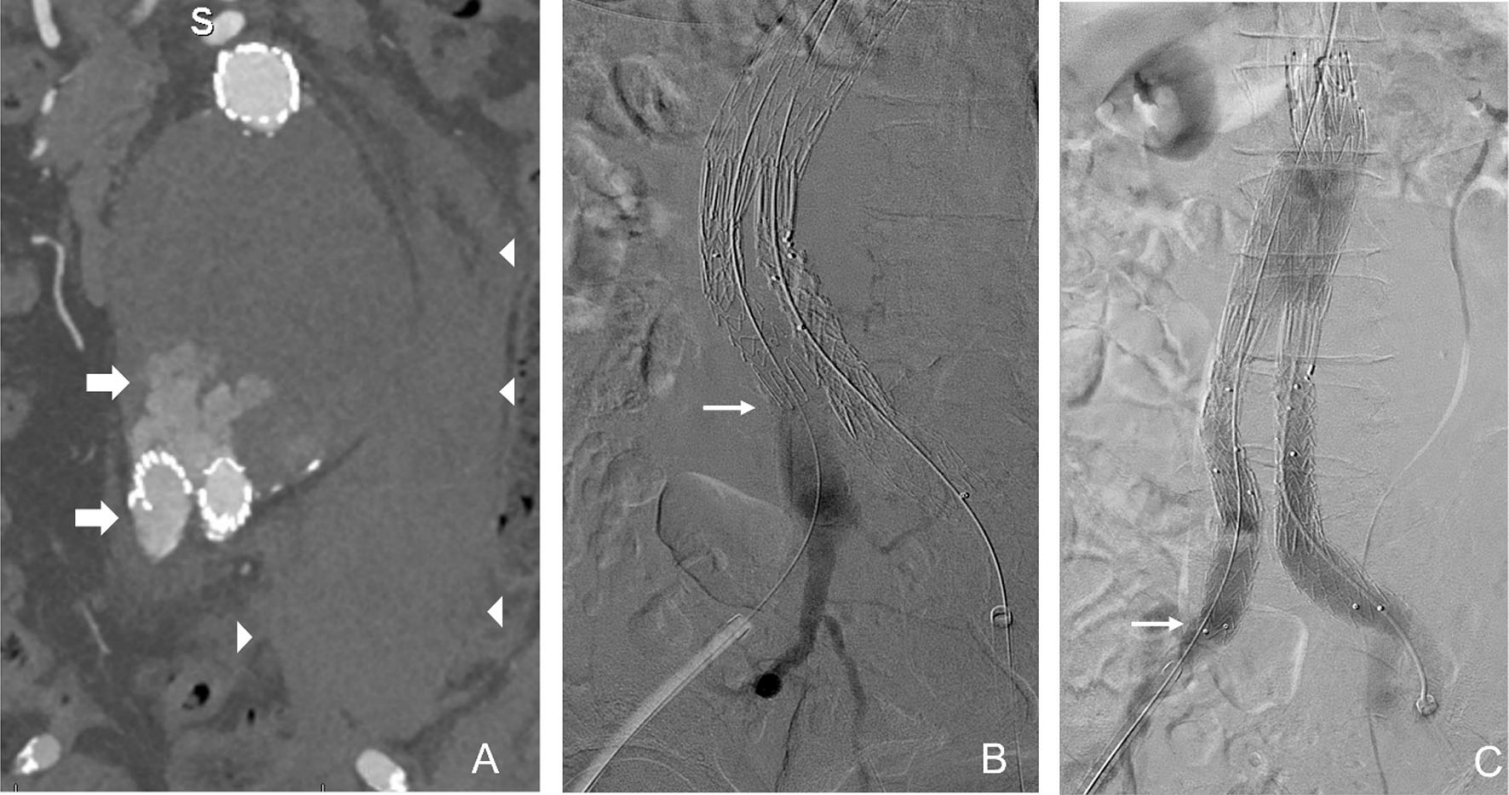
Type IB EL. (A) Coronal CT image shows a large type IB EL from a short right iliac limb (arrow). Patient presented with aneurysm rupture (arrowheads). (B, C) Angiograms before and after successful limb extension. Arrows point to distal extent of right iliac limb
